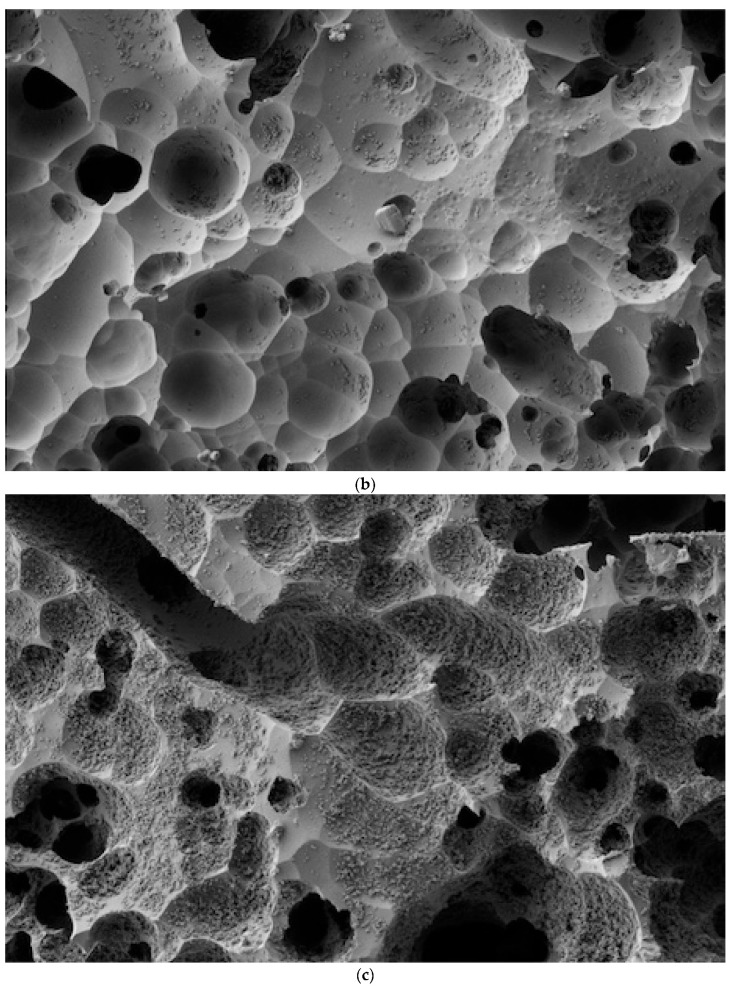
(a) The Calibra Try-in Paste cleaned with the Rinse and Dry method showed a very high amount of residual paste, occupying more than 75% of the image frame. (b) The Calibra Try-in Paste cleaned with Rinse and Dry followed by an Ultrasonic Bath in Distilled Water left a low amount of residual paste, covering less than 25% of the image frame. (c) The Calibra Try-in Paste cleaned with Rinse and Dry followed by an Ultrasonic Bath in Alcohol exhibited a very high amount of residual paste, similar to Rinse and Dry, occupying more than 75% of the image frame.
